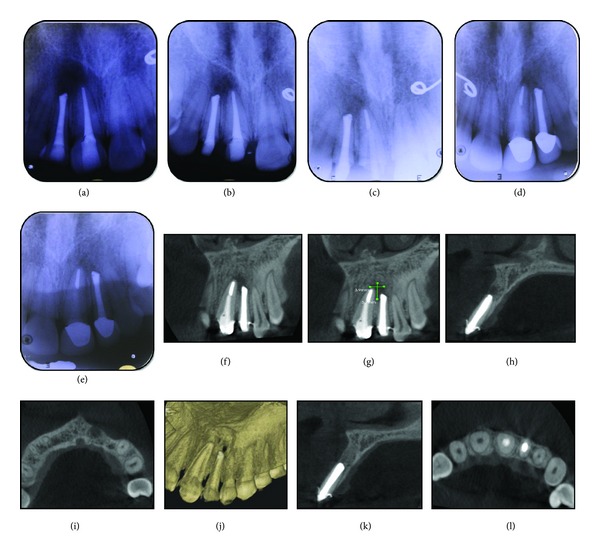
(a) IOPA radiograph at one month. (b) IOPA radiograph at three months. (c) IOPA radiograph at six months. (d) IOPA radiograph at one year. (e) IOPA radiograph at two-year followup. (f) Coronal plane CBCT image showing decreased size of periapical lesion at six months. (g) Coronal plane CBCT image showing measurement of periapical lesion; dimensions 5.7 mm/3.9 mm. (h) Sagittal plane CBCT image showing decrease in size of periapical lesion at six months. (i) Axial plane CBCT image showing decrease in size of periapical lesion at six months. (j) Three-dimensional CBCT image showing periapical lesion six months. (k) Sagittal plane CBCT image showing status of MTA obturation at six months. (l) Axial plane CBCT image showing status of root-canal filling at six months.
